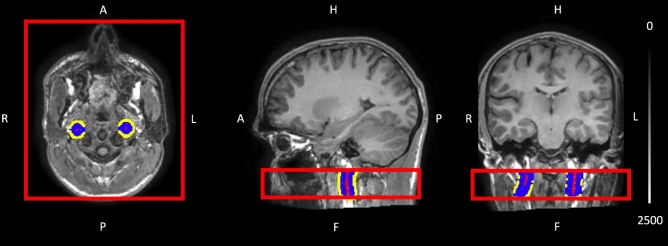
3D T1-weighted MR image with 3D MR angiography overlay (FOV shown in red) in MNI space, showing left and right internal carotid arteries (red), spill-over regions (blue) and background regions (yellow) and used to calculate an image derived input function.
